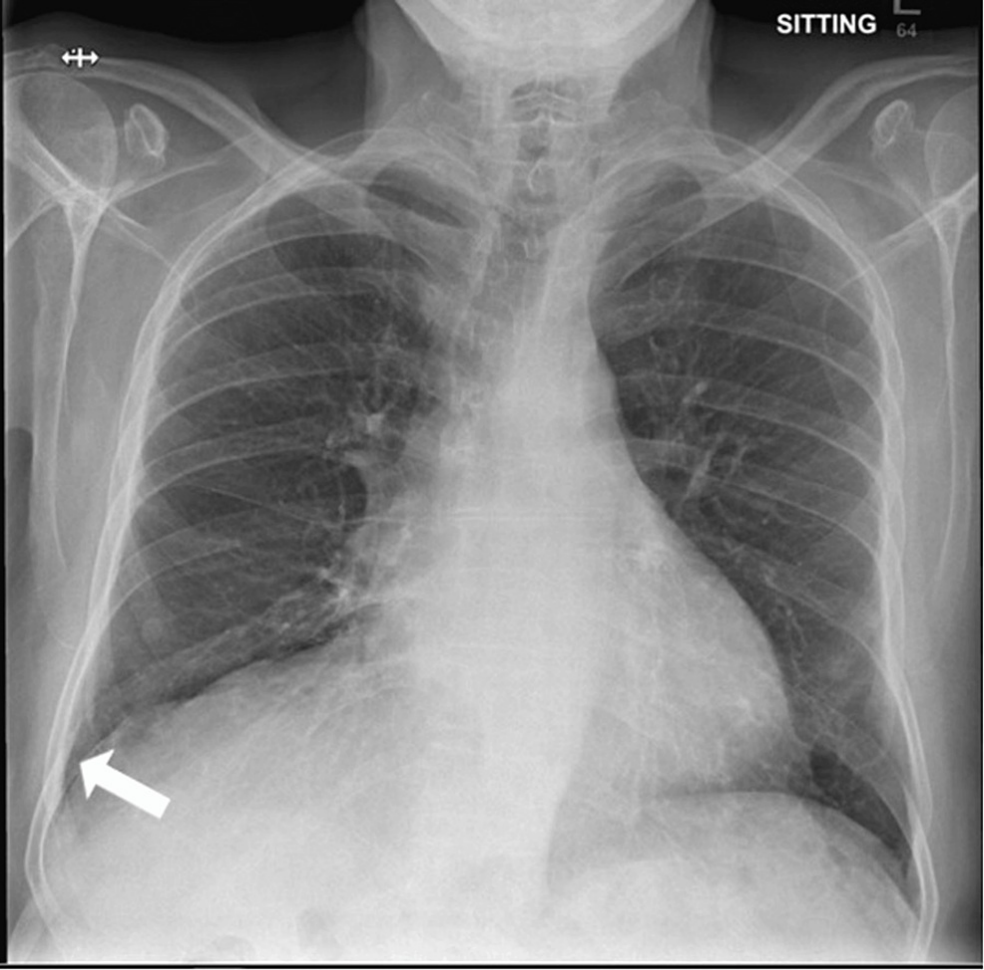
Chest X-ray from October 2020 showing right lower lobe consolidation or atelectasis and a small right-sided pleural effusion (white arrow). The left costophrenic angle appears clear, with no evident signs of pneumothorax.
